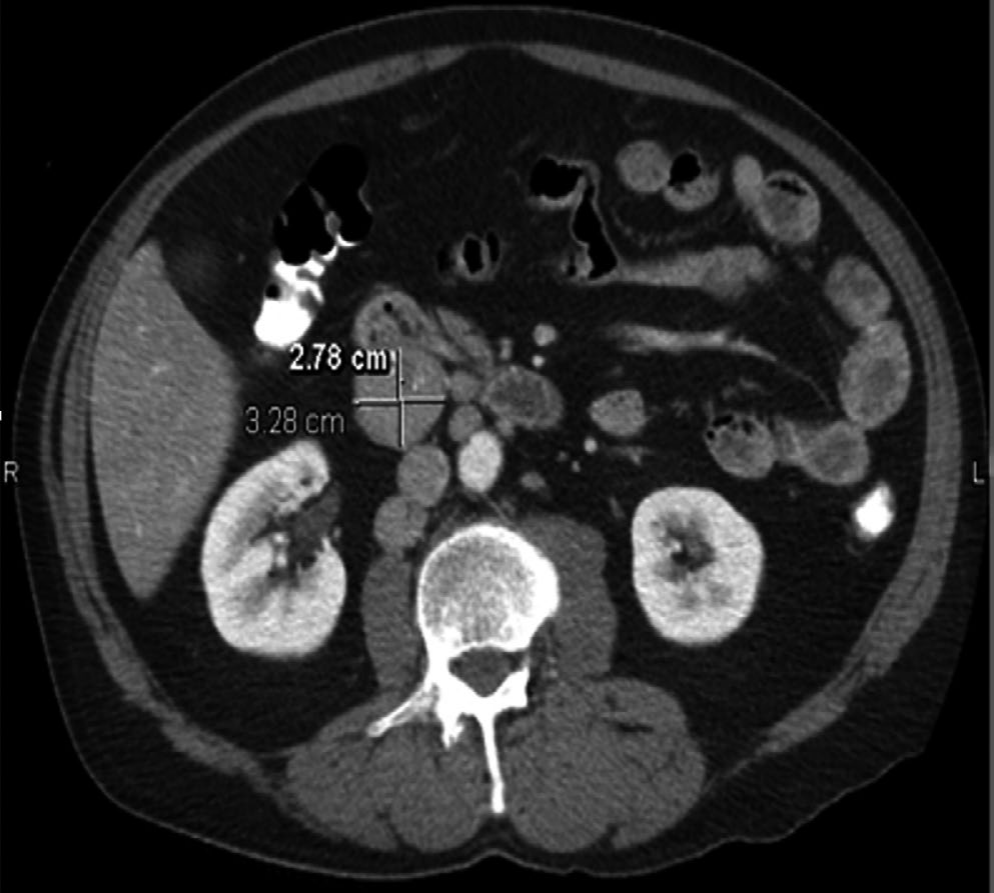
Computed tomography of abdomen showed a 2.8 × 3.3 × 3.5 solid partially calcified right retroperitoneal mass arising from the pancreatic head/duodenum and associated mild retroperitoneal lymphadenopathy
